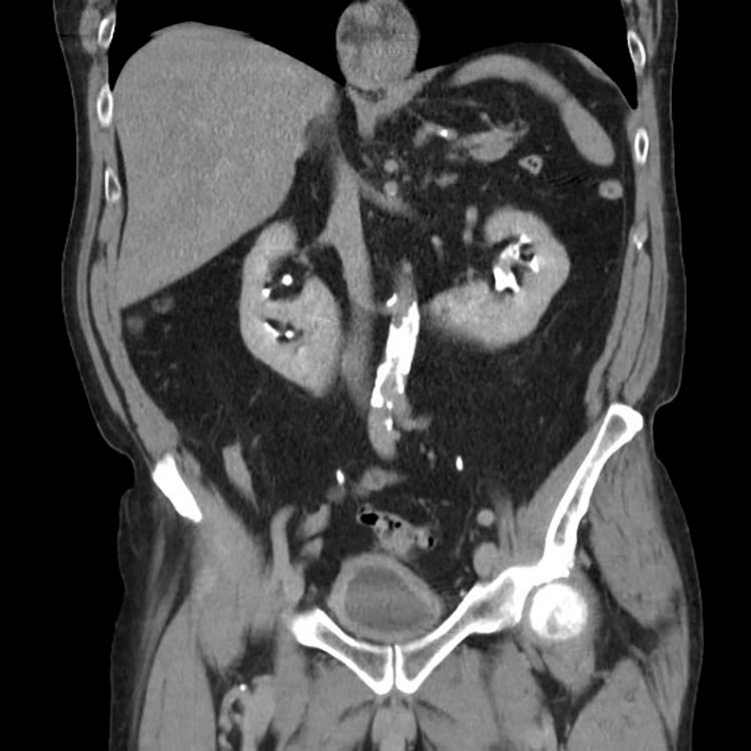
Coronal CT (urographic phase) demonstrating filling defect left upper pole.
